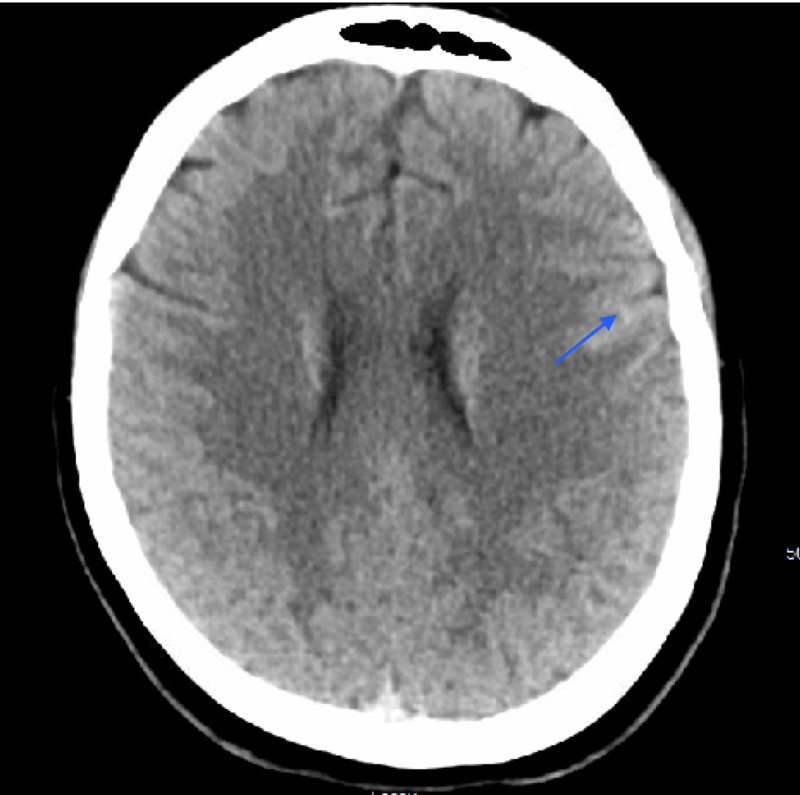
Axial image of CT head showing a hyper-intense signal in the left frontal convexity CT: computed tomography
